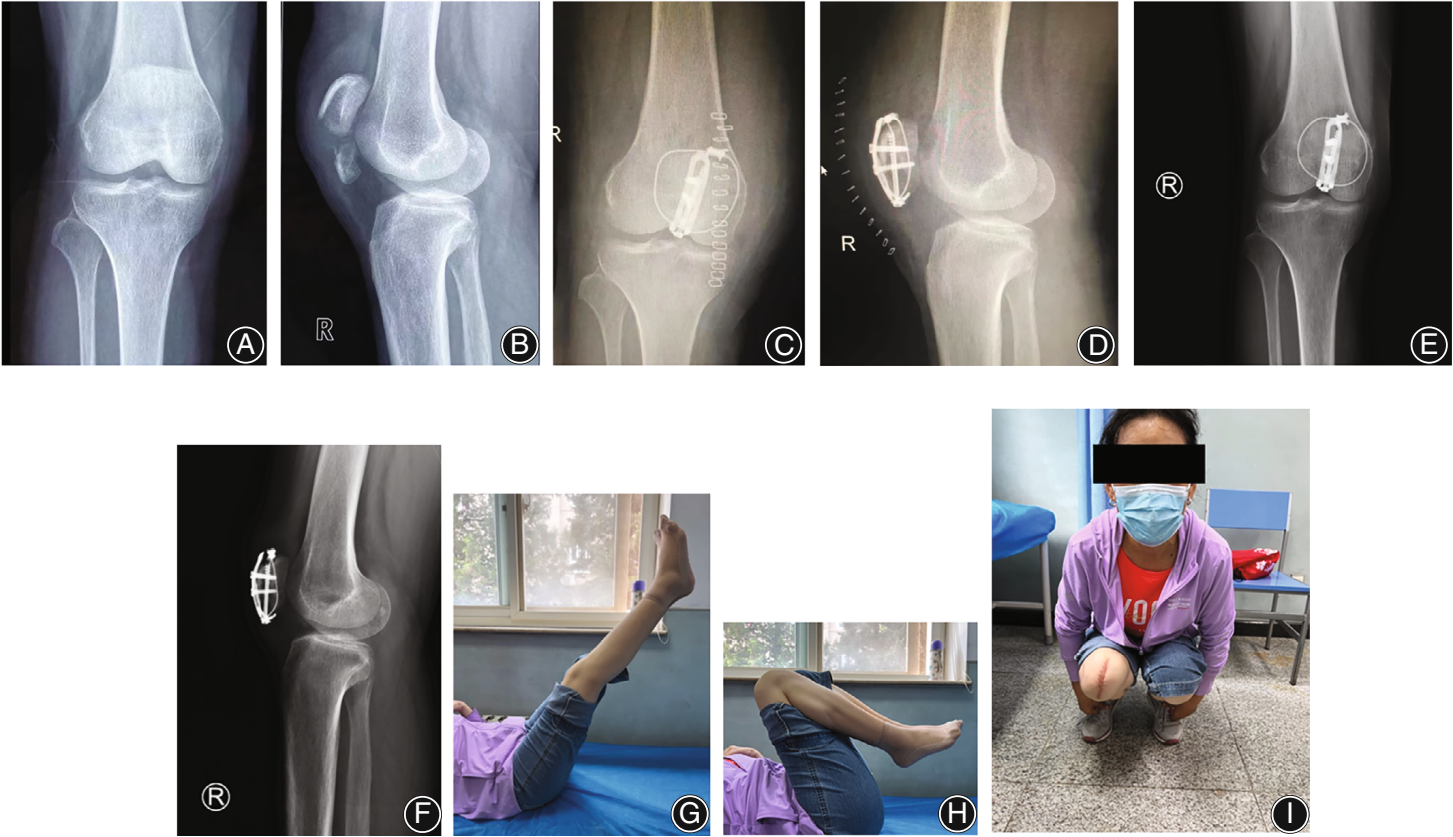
Case example (case 10, 45 years, female, right side). Preoperative anteroposterior (A) and lateral (B) radiographs showed a displaced inferior patellar pole fracture. Postoperative anteroposterior (C) and lateral (D) radiographs. Radiographs (E, F) and function (G–I) at 19 months follow‐up
